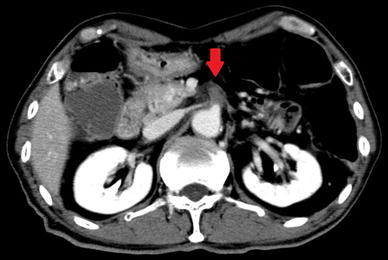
Contrast-enhanced computed tomography showed occlusion 3 cm across from the origin of the SMA (*down arrow*)
